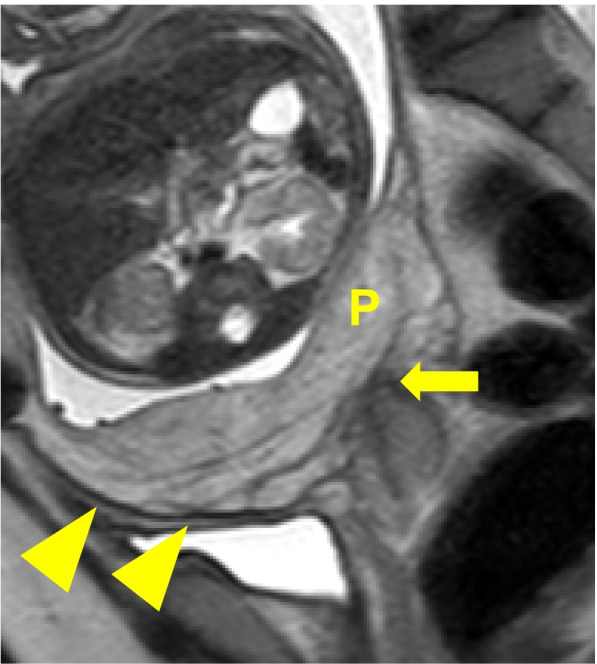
Sagittal T2-weighted magnetic resonance image at 33 weeks’ gestation. The thin hypointense interface between the placenta and the myometrium is preserved (arrowheads). The internal cervical os is indicated by an arrow. P: placenta
